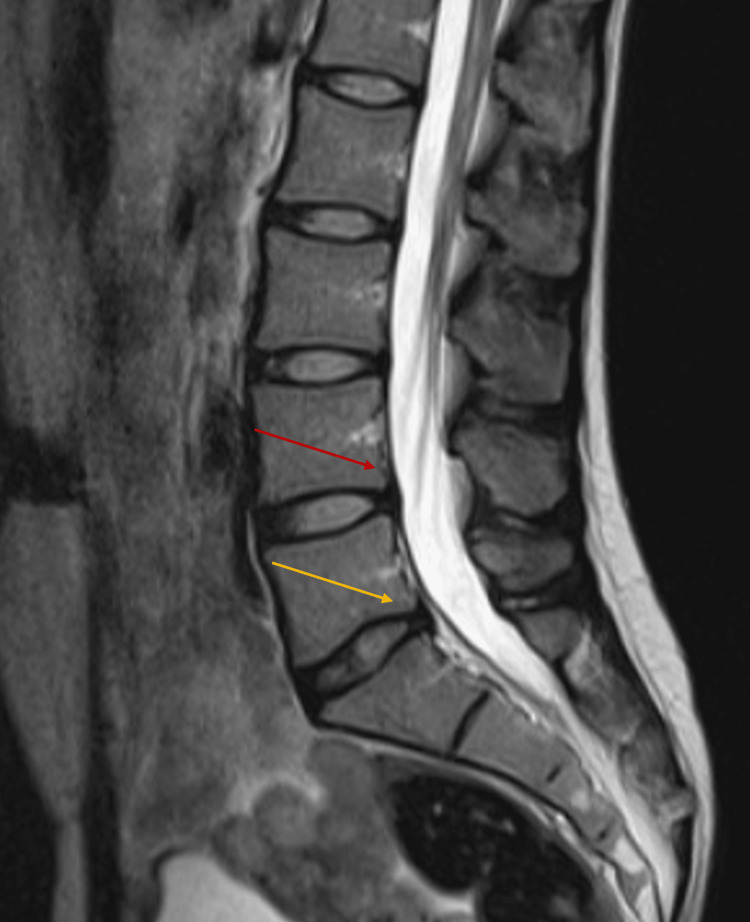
Saggital MRI of the lumbar spine showing mild dorsal protrusion of the spinal disc at L4-L5 (red arrow) and L5-S1 (orange arrow). MRI: magnetic resonance imaging
